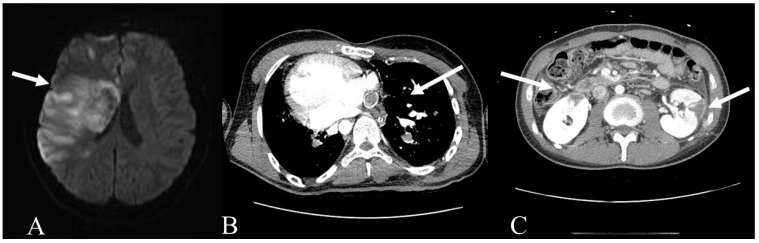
Multiple embolic events occurred in our patient, including (A) acute right middle cerebral artery territory infarction on magnetic resonance imaging, (B) pulmonary thromboembolism, and (C) multifocal renal infarctions on computed tomography.
